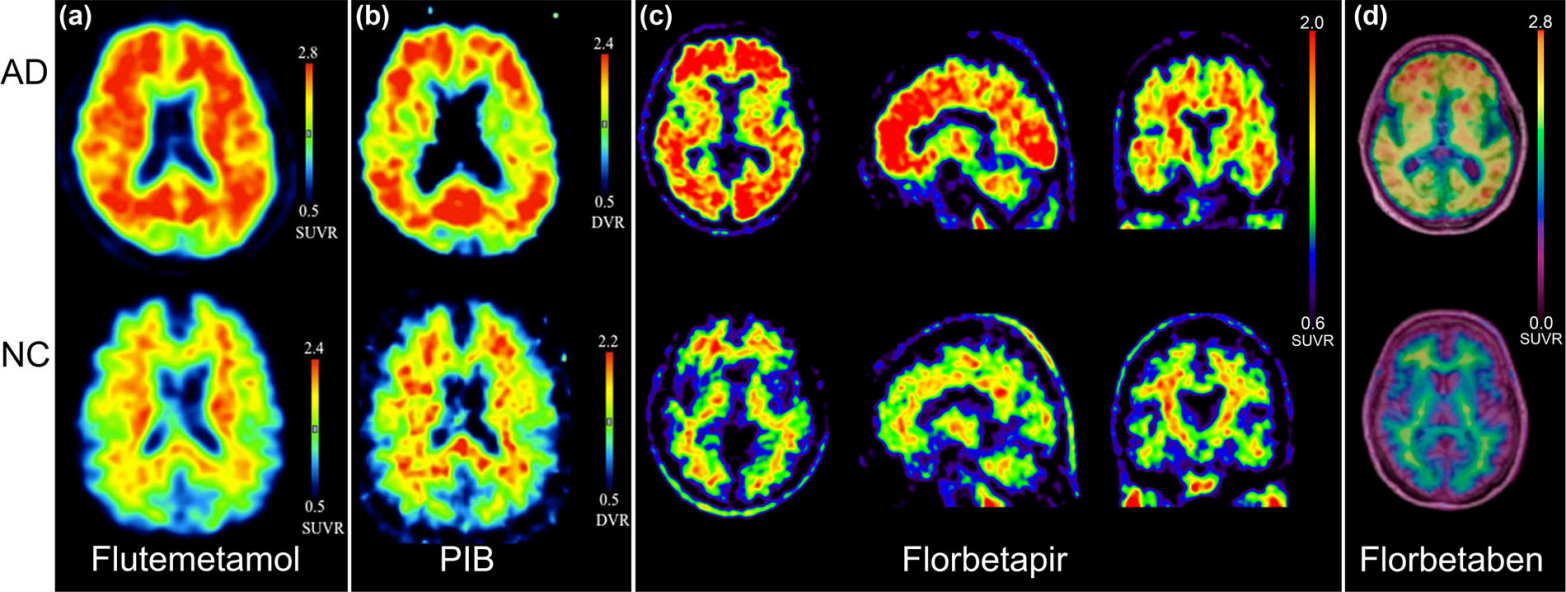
The typical brain images of Alzheimer's disease (AD) and normal controls are shown by amyloid‐β (Aβ) PET imaging. Part (a) shows axial ^18^F‐flutemetamol (^18^F‐FMM) PET images (upper row for AD patients, lower row for normal controls). Part (b) shows axial ^11^C‐PIB PET images (upper row for AD patients, lower row for normal controls). Part (c) shows ^18^F‐AV45 PET images in transaxial, sagittal, and coronal positions (upper row for AD patients, lower row for normal controls). Part (d) shows axial ^18^F‐florbetaben PET images with aligned fused MRI images (upper row for AD patients, lower row for normal controls). *Source*: From Camus et al. (2012, Hatashita et al. (2014)), and Villemagne et al. (2011) with modifications
